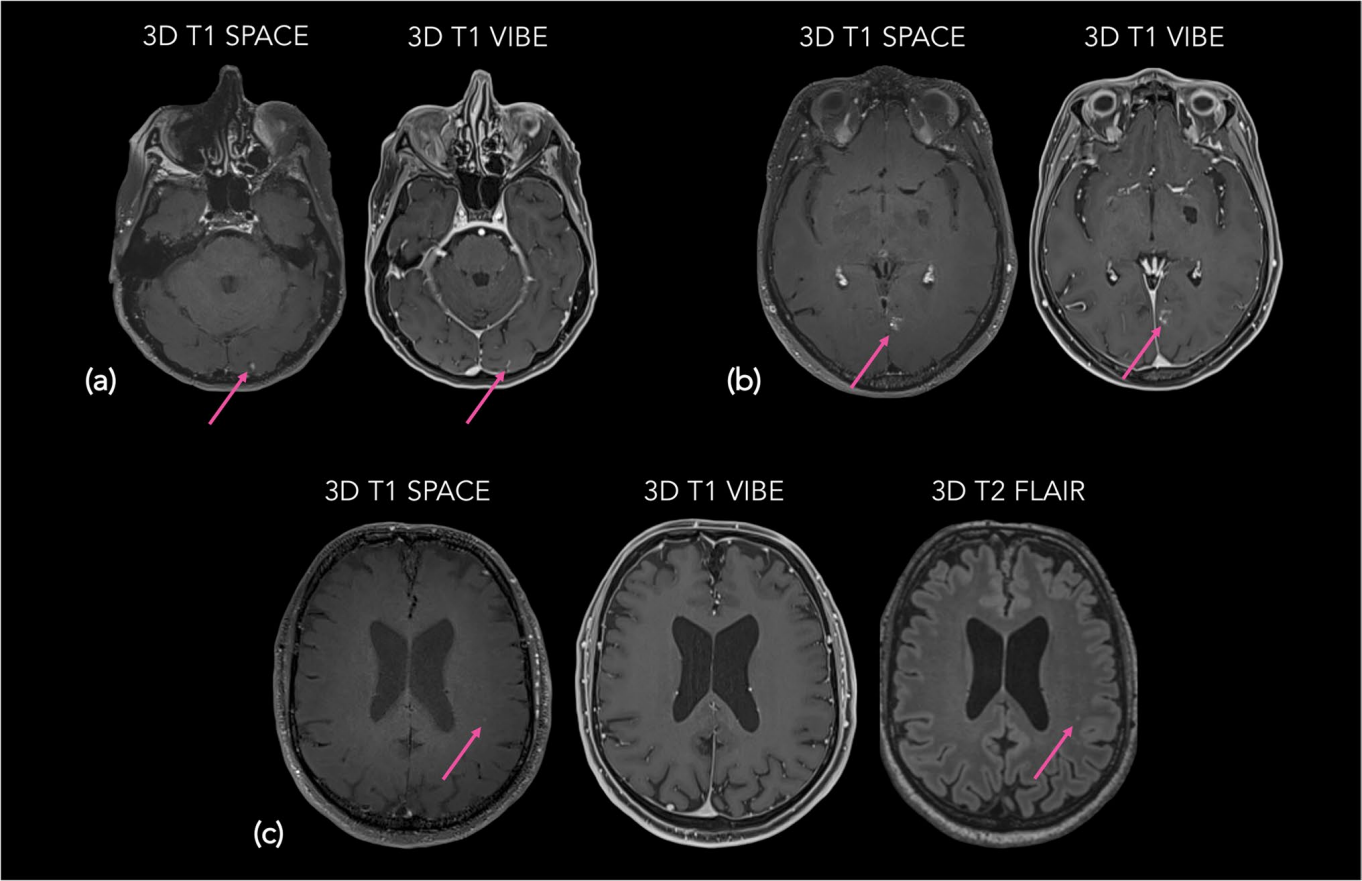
Examples of false positive cases of SPACE in detecting brain metastases demonstrated by CE axial slices. a Non-suppressed small left occipital cortical vessel (arrow) appearing on SPACE (left) as an enhancing lesion, better discernible on VIBE (right) in a 70-year-old female with lung cancer. b Non-suppressed left parasagittal developmental venous anomaly (arrow) appearing on SPACE (left) as an enhancing lesion, better discernible on VIBE (right) in a 62-year-old female with lung cancer. c Left periventricular white matter hyperintensity (arrow) appearing on SPACE (left) as an enhancing lesion; confirmed in T2w FLAIR sequence (right); no enhancing area is visible on VIBE (middle) in a 65-year-old male with lung cancer. SPACE, sampling perfection with application-optimized contrasts by using different flip angle evolutions; CE, contrast-enhanced; VIBE, volumetric interpolated breath-hold examination; T2w, T2 weighted; FLAIR, fluid attenuated inversion recovery; 3D, three dimensional
